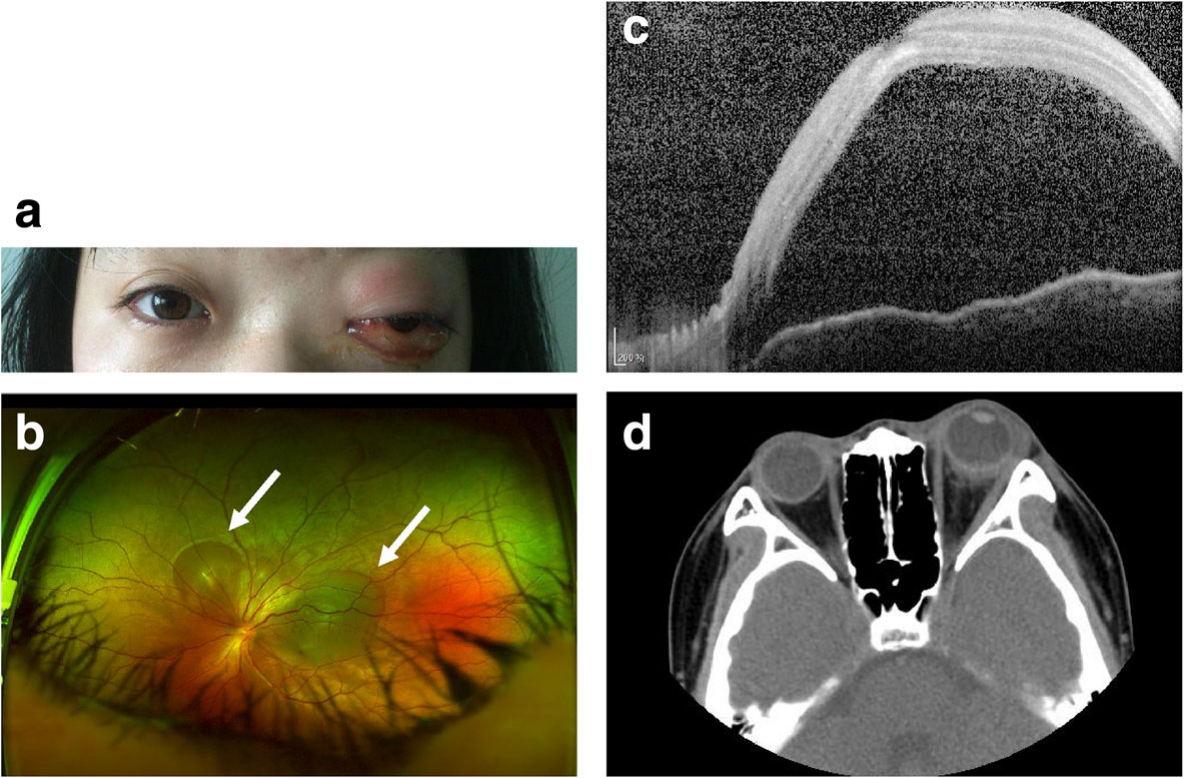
a The patient showed erythema and edema of the eyelids and chemosis. b Color fundus photograph revealed two ERD lesions that were temporal and superior-nasal to the optic disk (arrows). The presence of left eye ERD was further confirmed by SD-OCT (c). d Orbital CT scans demonstrated left eye proptosis, swelling of the periorbital and postbulbar soft tissue, and the presence of ERD (arrow)
